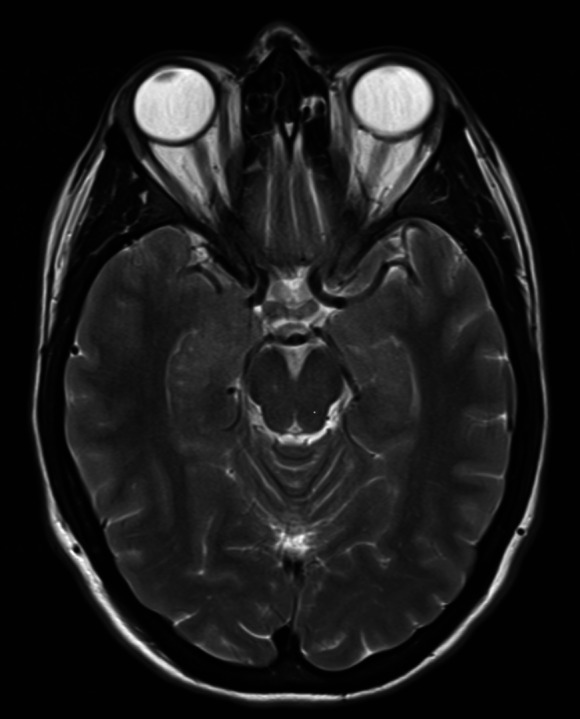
Pre-contrasted T2-weighted MR image (acquired at symptom onset) from a 31-year-old seropositive woman who presented with altered mental status and seizures. Enlarged oedematous hippocampus.
